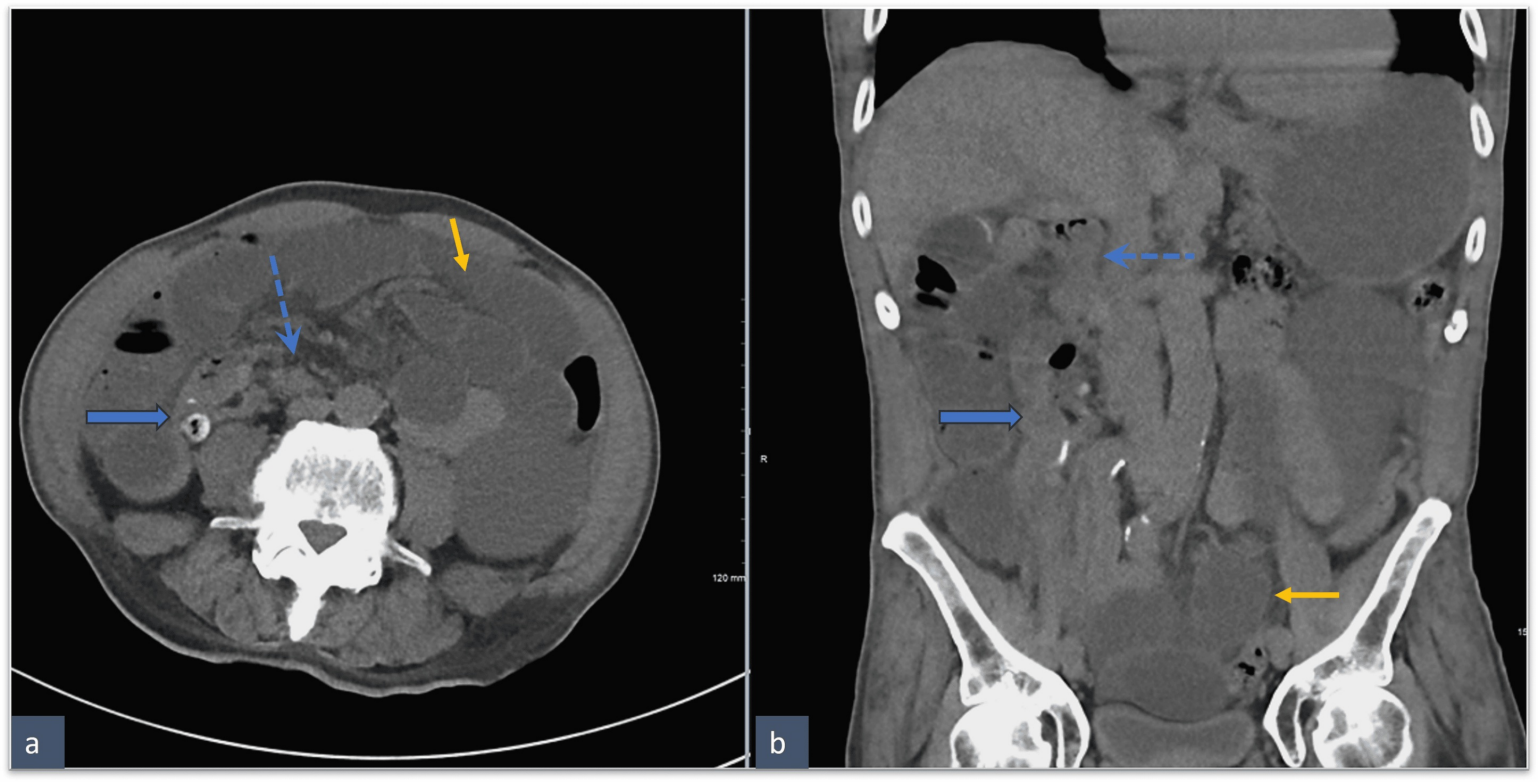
Non-contrast CT of the abdomen and pelvis at presentation Dilated small bowel loops are compatible with high-grade obstruction marked by the solid yellow arrow. The blue dashed arrow marks the duodenum. The blue block arrow marks the cecum with a diverticulum. CT: computed tomography
